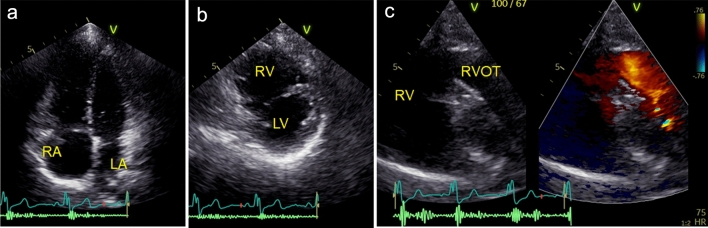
A case of severe pulmonary regurgitation following intracardiac repair for tetralogy of Fallot. a Apical four-chamber view. b Parasternal short-axis view. c Severe pulmonary regurgitation in RV outflow view. Dilatation of the RV relative to the LV is observed. Although the pulmonary valve is not visualized clearly enough to assess its characteristics, pulmonary regurgitation with wide jet convergence is observed. *LA* left atrium, *LV* left ventricle, *RA* right atrium, *RV* right ventricle, *RVOT* right ventricular outflow tract
